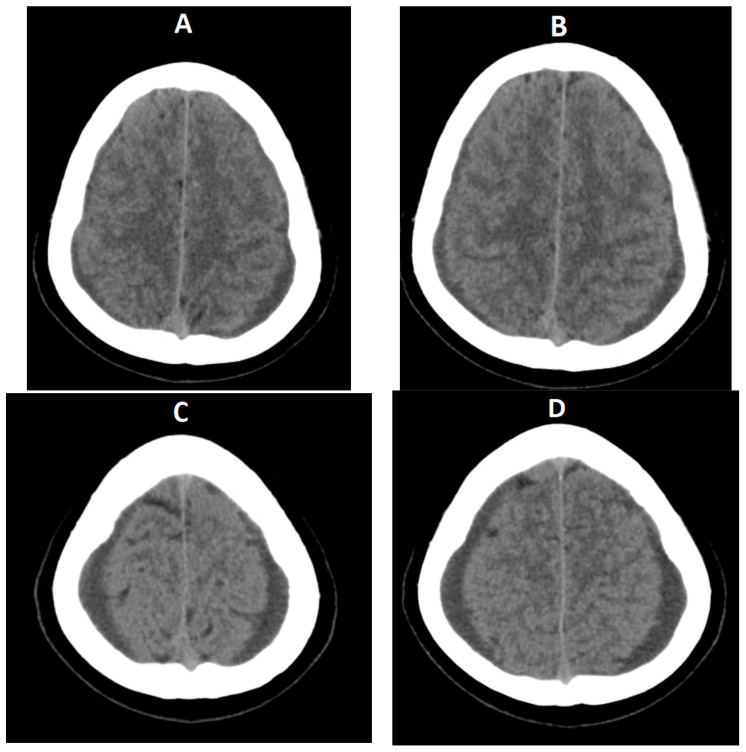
CT scan on third admission (A,B) and one week later of the patient (C,D) showing enlarging bilateral temporo-parietal subdural hygromas measuring 5 (A,B) and 10 mm (C,D).
